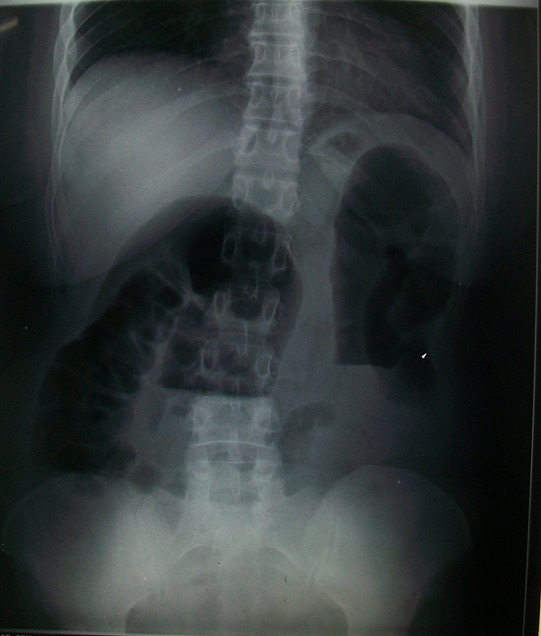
Abdominal X-ray showing the presence of air-fluid levels
